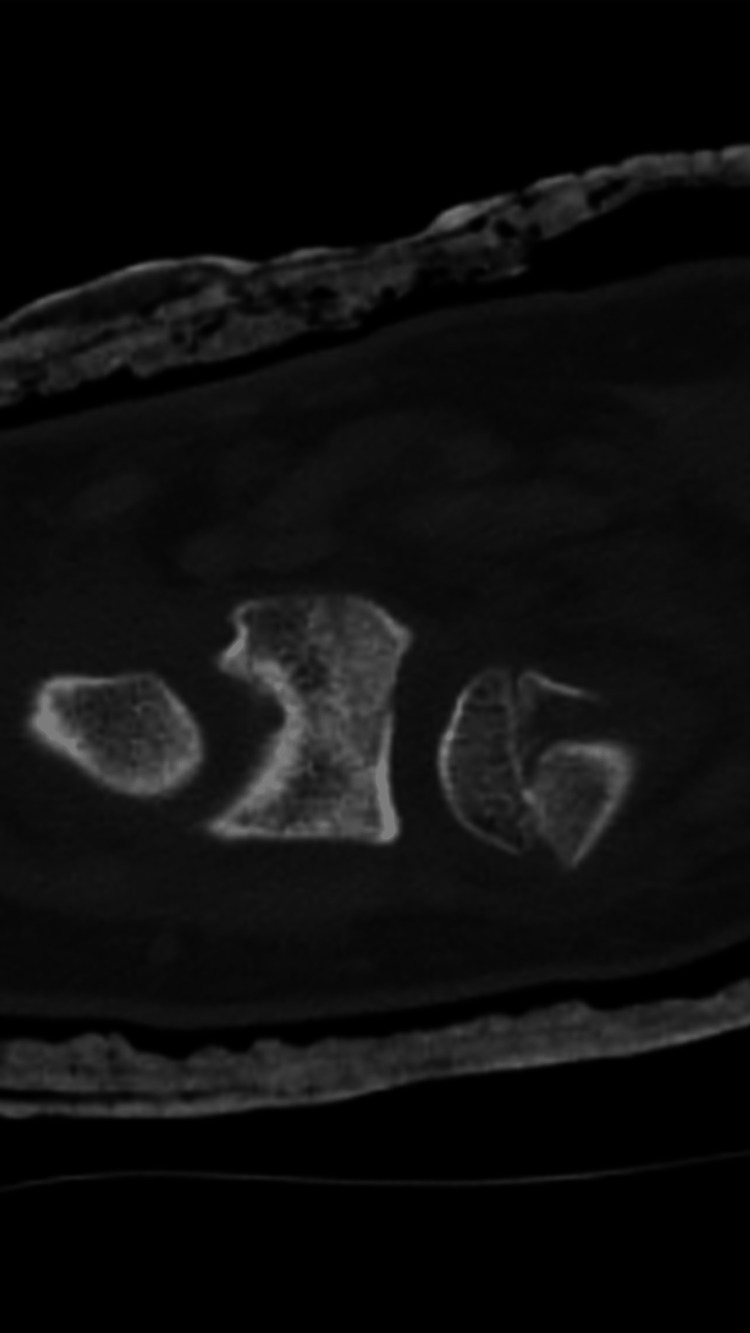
CT imaging of the left wrist in the sagittal plane. CT: computed tomography.
